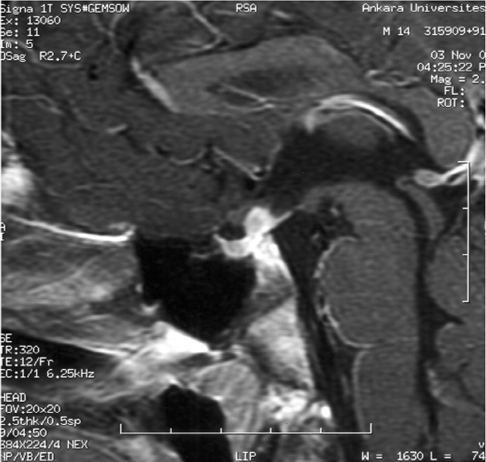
MRI at follow−up: Enlargement of PST from 5 mm to 7 mm
